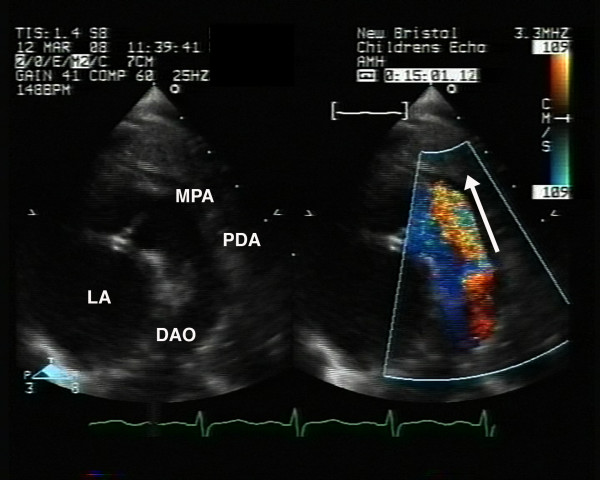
Echocardiography, a high parasternal long axis view demonstrating a large patent duct (PDA). Colour flow demonstrates shunting from 'left to right', from the descending aorta (DAO) to the pulmonary arteries (MPA).
